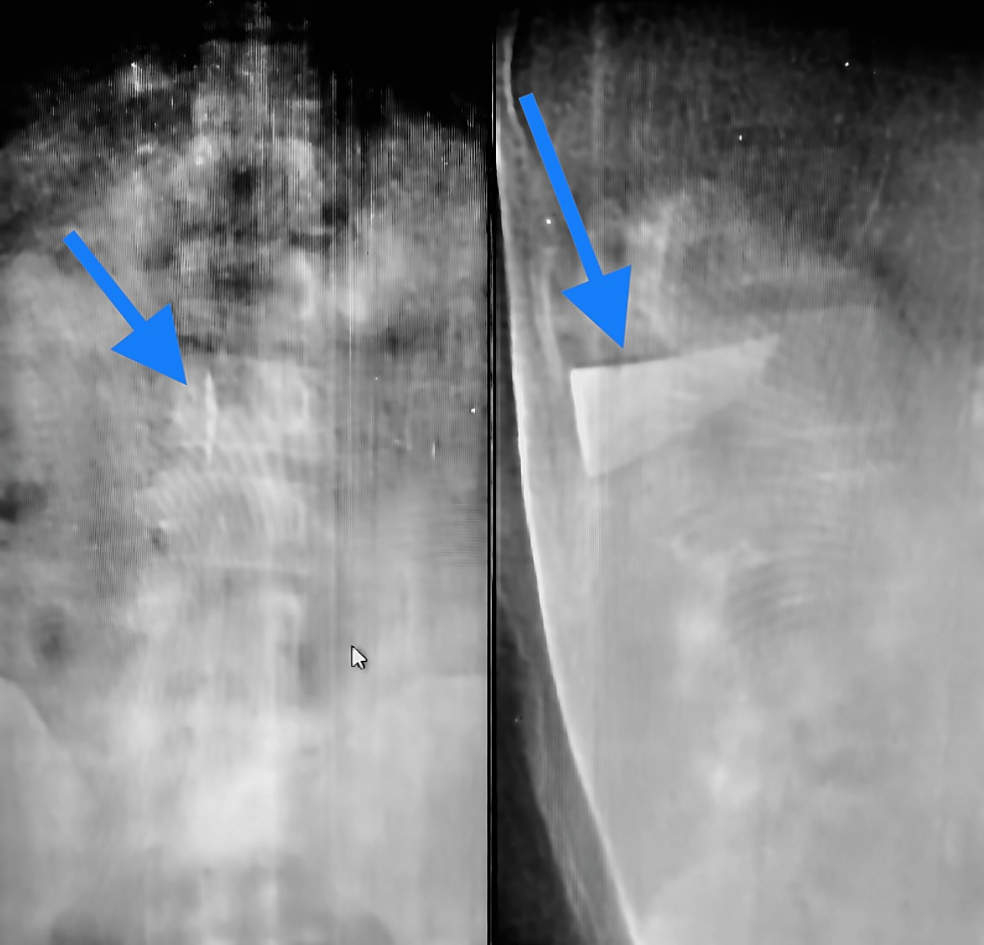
Foreign body on X-rays Antero-posterior and lateral X-ray view showing foreign body (knife blade) marked with a blue arrow
